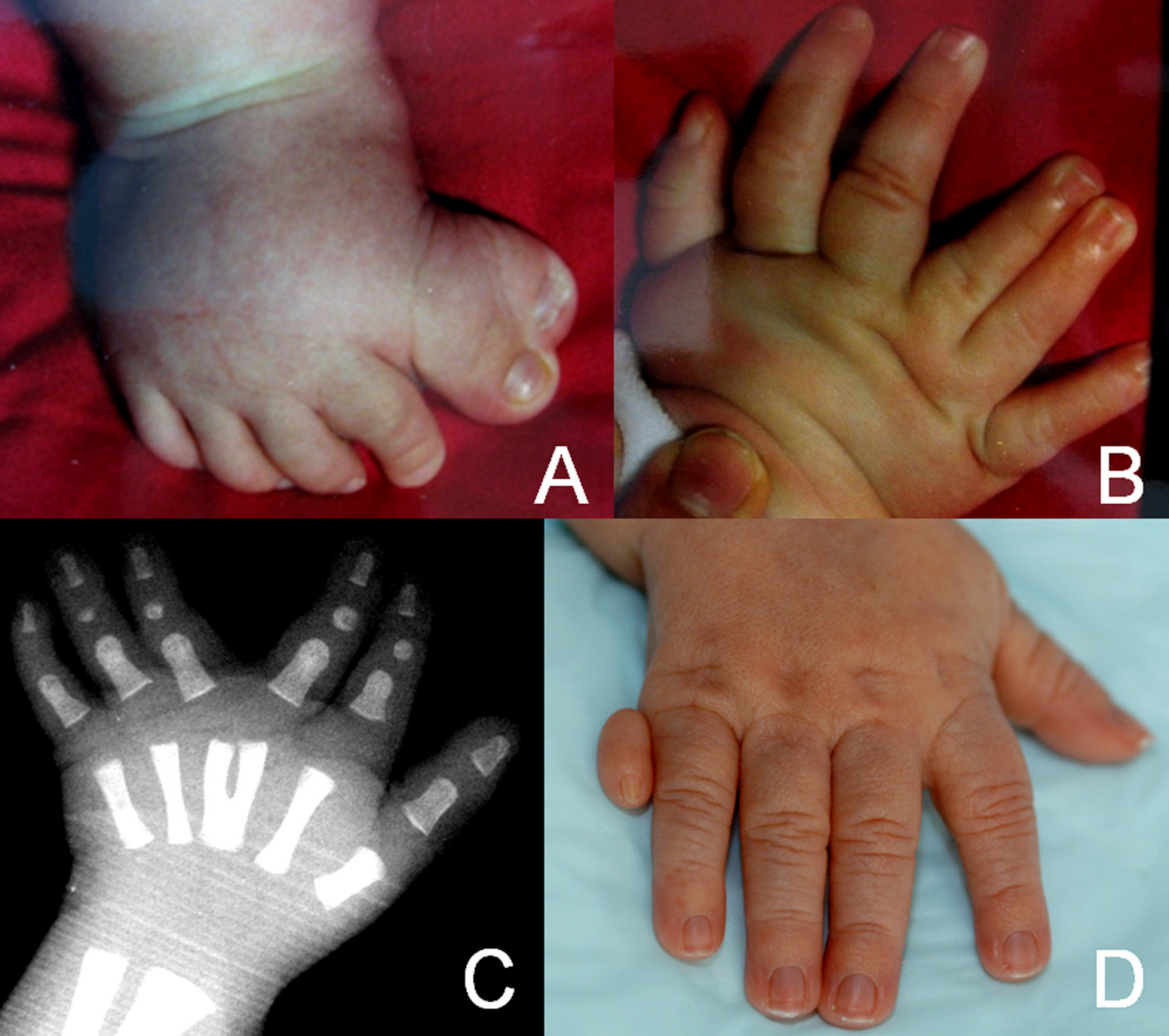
A, preaxial poly-syndactyly of the right foot, B, mesoaxial polydactyly of the right hand, C, X-ray of the left hand showing a Y-shaped third metacarpal bone, and D, postaxial polydactyly of the right hand.
